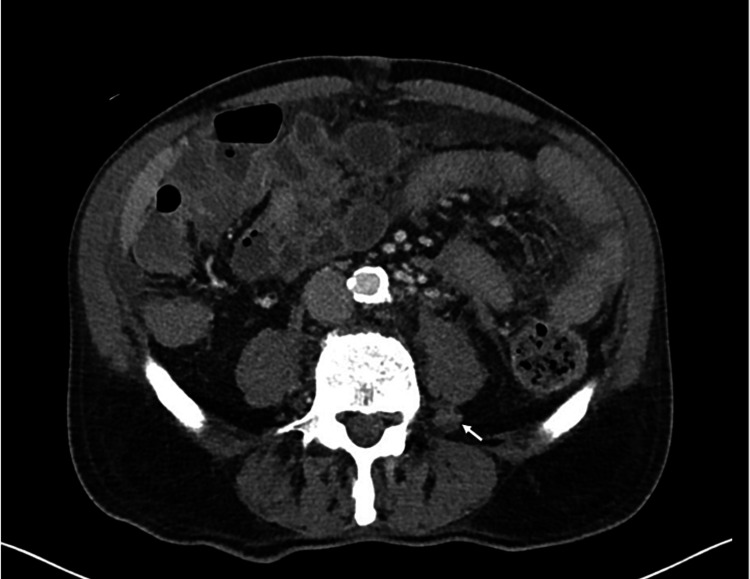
CT scan of the abdomen showing retroperitoneal lymphadenopathy (white arrow shows lymphadenopathy adjacent to the left psoas muscle) CT: computed tomography
